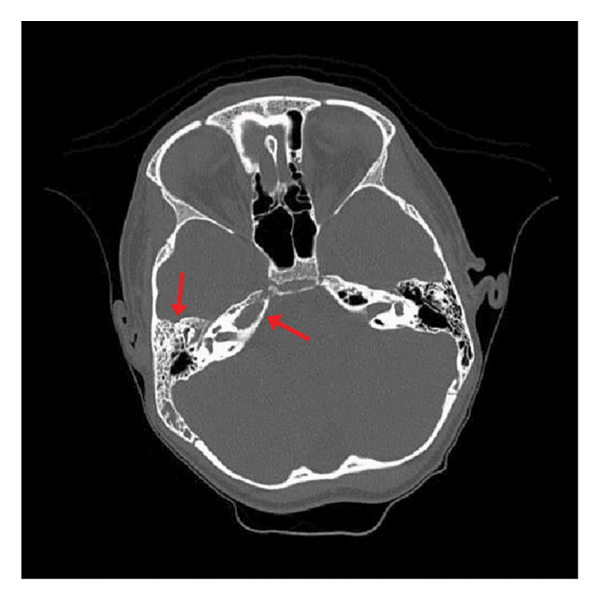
CT temporal bone on admission demonstrating right mastoid and middle ear opacities, predominantly within the epitympanum, with mild thinning of the tegmen tympani and tegmen mastoideum. No discrete ossicular erosion was observed. Pneumatization of the bilateral petrous apices with opacification of the right petrous apex is shown.
